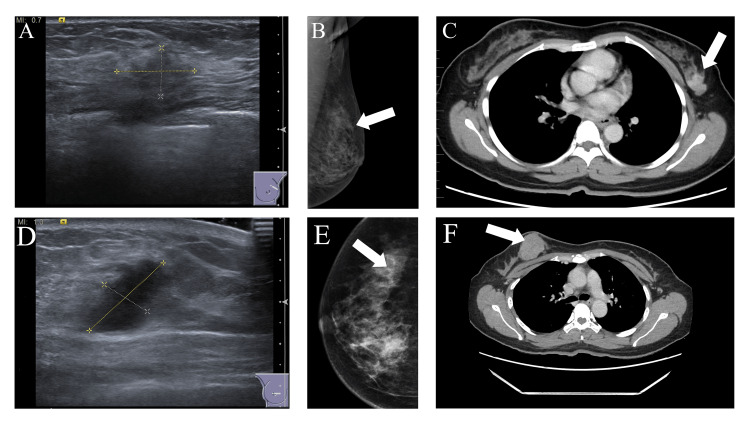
Radiology findings of both the left and right sides (A) Gray-scale ultrasound shows an irregular hypoechoic lesion at 2 o'clock on the left breast. (B) The left mediolateral oblique (MLO) mammogram shows an equal-density mass with speculated margins in the upper outer quadrant of the left breast. (C) Contrast-enhanced CT scan of the chest shows an enhancing soft tissue lesion with irregular margins at the upper outer quadrant of the left breast. (D) Gray-scale ultrasound shows a hypoechoic lesion with an irregular margin, taller than wider with surrounding architectural distortion at 1 o'clock on the right breast. (E) The right CC mammogram shows an irregular, dense, spiculated mass lesion in the right inner quadrant. (F) Contrast-enhanced CT scan of the chest shows a well-defined, heterogeneously enhancing lesion with a necrotic component associated in the upper inner quadrant of the right breast with enlarged metastatic axillary lymph nodes.
